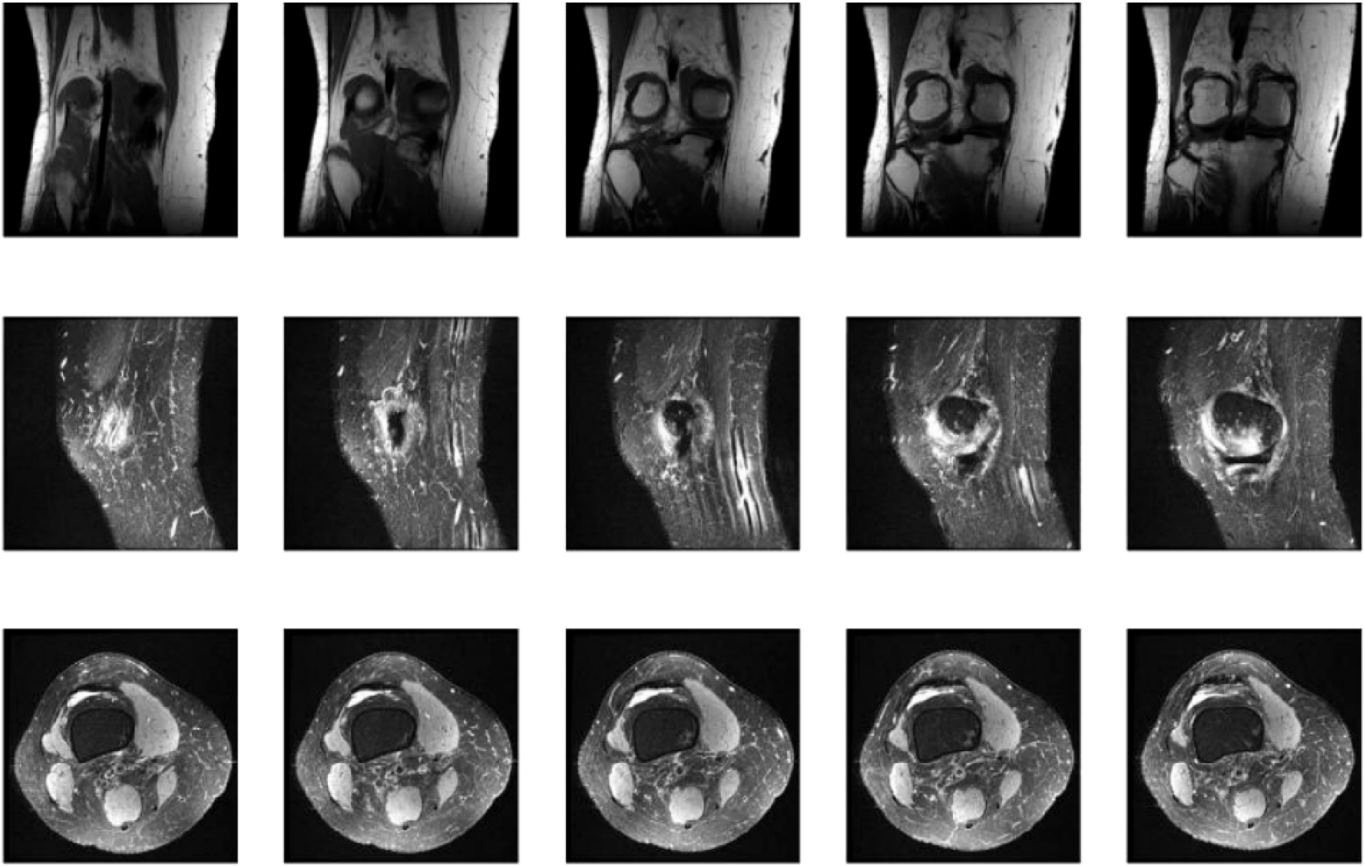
Representative coronal, sagittal, and axial MRI views of the knee joint, providing complementary information for the comprehensive assessment of knee anatomy and pathology.
